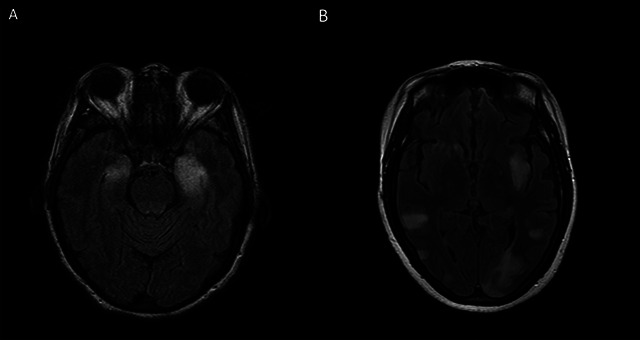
Axial fluid-attenuated inversion recovery (FLAIR) (A,B). A. Increased signal on both medial temporal lobes in a patient with Limbic encephalitis associated with-anti AMPAR antibodies; B. multiple cortical and subcortical FLAIR signal changes involving both hemispheres, without restriction on diffusion sequences in a patient with GABAaR encephalitis Gamma-Amino Butyric Acid type A receptor alpha-amino-3-hydroxy-5-methyl-4-isoxazolepropionic acid receptor.
